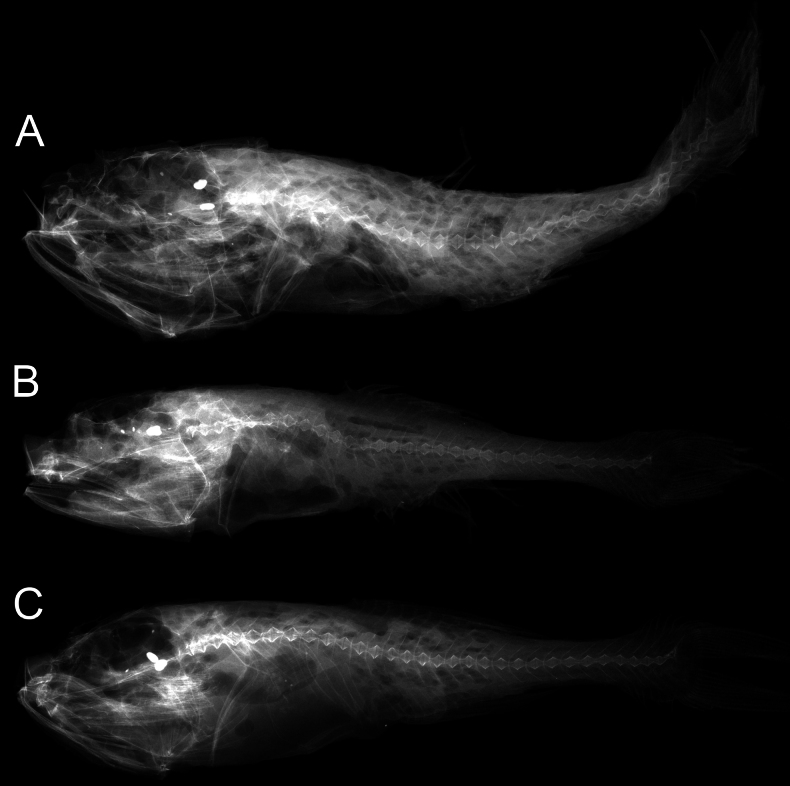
X-radiographs of *Malacosarcusmacrostoma* (Günther, 1878) AASIZP 73637, 65.5 mm SLBASIZP 73644, 55.7 mm SLCASIZP 73646, 61.2 mm SL.
